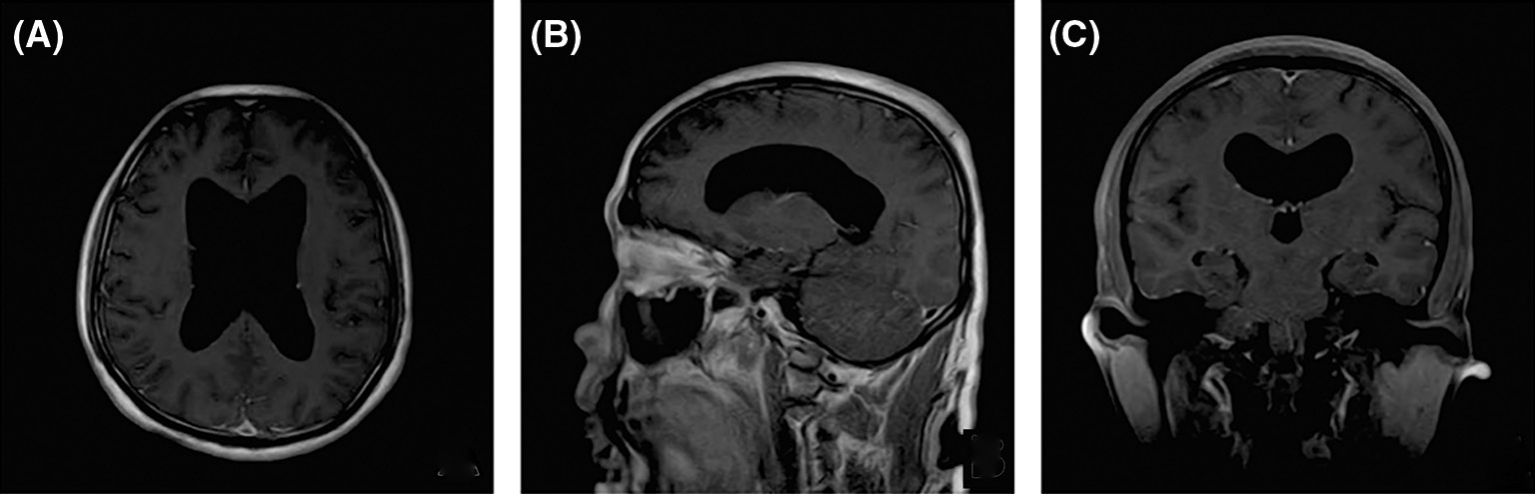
Findings of brain magnetic resonance imaging. Panel A: axial strengthening; Panel B: sagittal enhancement; and Panel C: coronal enhancement. The panels show supratentorial hydrocephalus.
